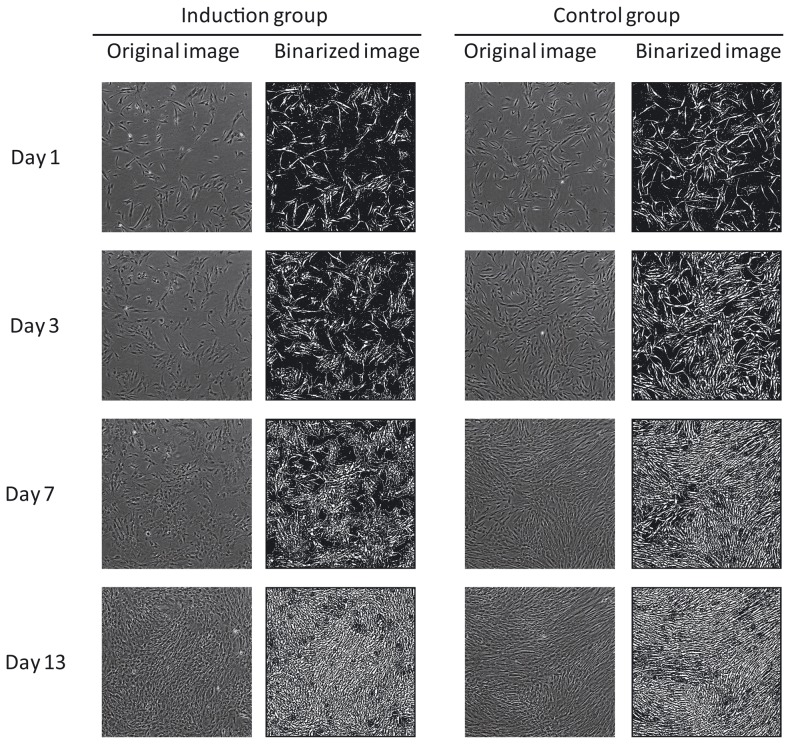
Phase contrast raw image from BioStation CT and its processed image. The images of beginning (day 1), middle (day 3 and 7), and the end (day 13) in the induction period of Lot 1 are indicated as examples. Raw images were binarized with MetaMorph.
